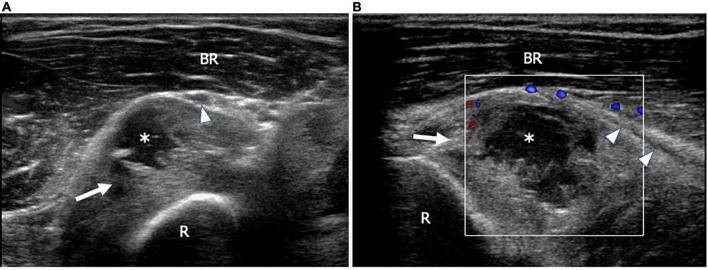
This figure shows two 18 MHz HRUS images (A,B) of a 53 years old male patient affected by rheumatoid arthritis with a progressive decrease of fingers extension strength. (A) An axial view, immediately distal to the humeral-radial joint line, shows the annular recess (white arrow) distended by synovium and fluid (white asterisk); the recess dislocates superficially the posterior interosseus nerve (white arrowhead) immediately proximal to the supinator tunnel. After switching on the Eco-Color Doppler, a slight peripheral vascularization of the synovial wall of the annular recess (white arrow) is shown (B). (B) The longitudinal scan also depicts the long axis of the posterior interosseous nerve (white arrowheads) dislocated superficially by the distended recess. BR, brachioradialis muscle; R, radius.
